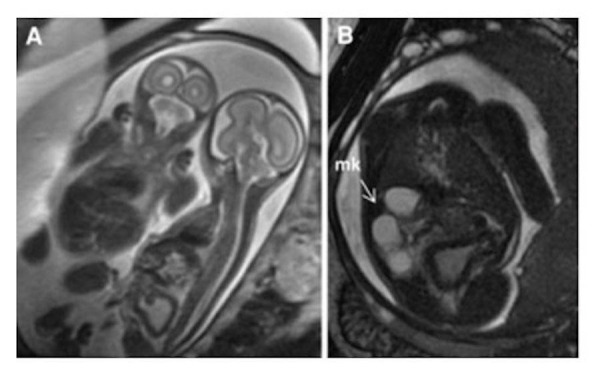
Multicystic kidney T2-weighted HASTE imaging of monochorionic, monoamniotic twins. Panel A demonstrates the affected twin is on the right with a thickened and distended bladder. Panel B is an axial slice demonstrating a multicystic kidney (arrow). Reprinted without change from Bischoff, et al. under the creative commons license
